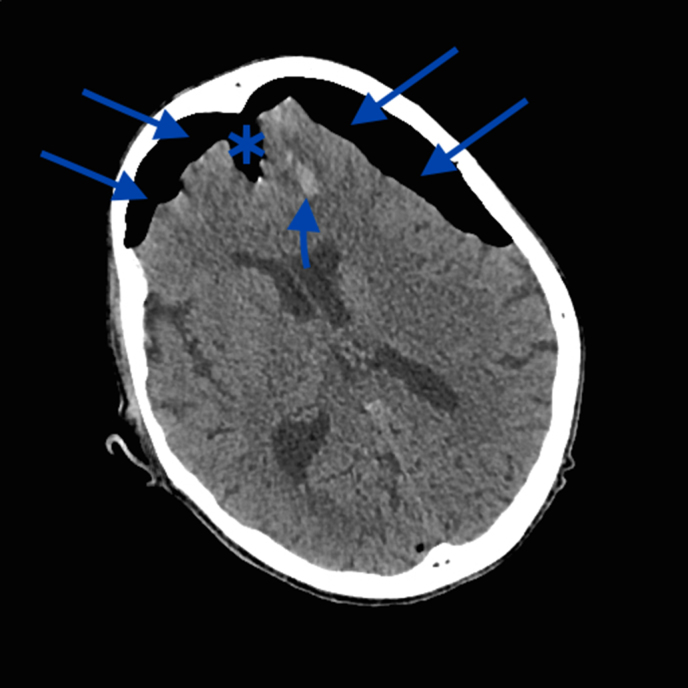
Unenhanced axial CT brain slice on brain windows demonstrating the “Mount Fuji” sign. There is a large volume of subdural air (long arrows) which is compressing and causing separation (star) of both frontal lobes away from the midline falx cerebri, pathognomic of tension pneumocephalus. A small heamorrhagic contusion (short arrow) is also seen.
